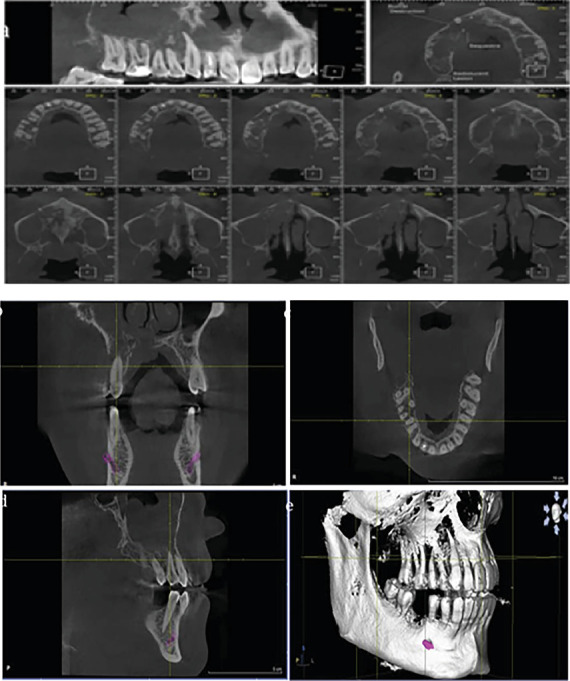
(a–e) Axial, sagittal, and coronal CBCT images and 3D reconstructed views showing severe bone destruction and sequestration.
